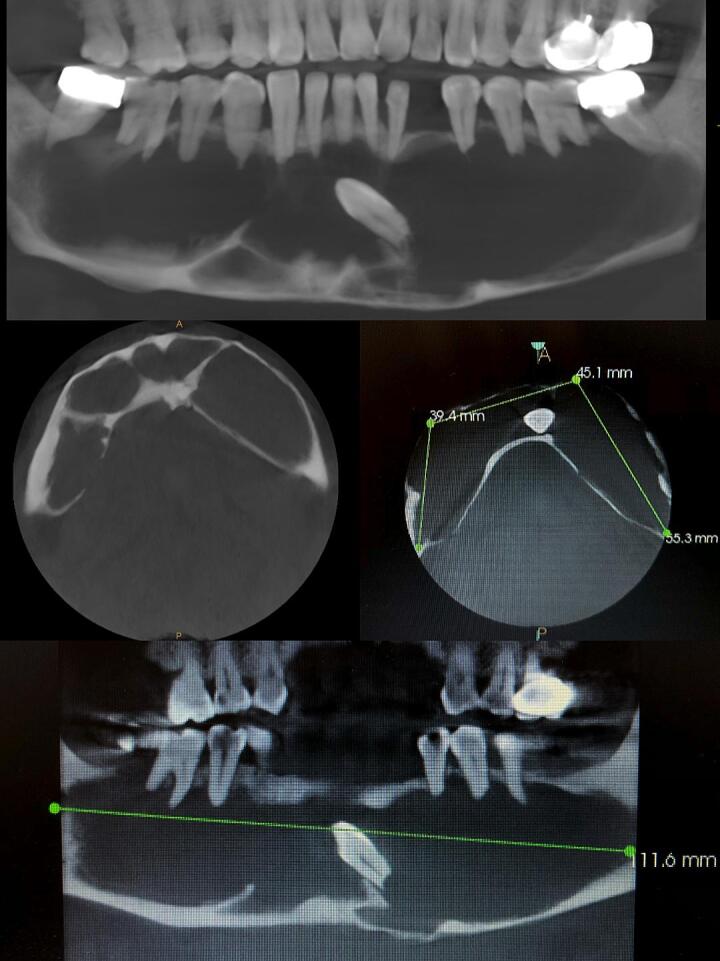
CT images reveal the invasive size and multi-chamber appearance of the lesion.
